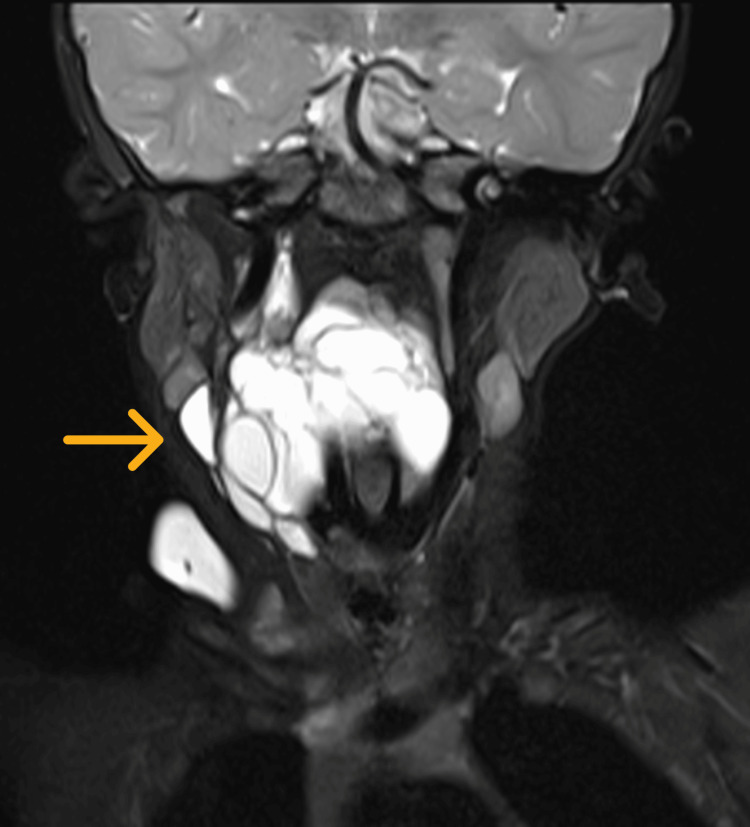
The same multiloculated cystic lesion shows a hyperintense signal (arrow) on an MRI neck T2-weighted image.
